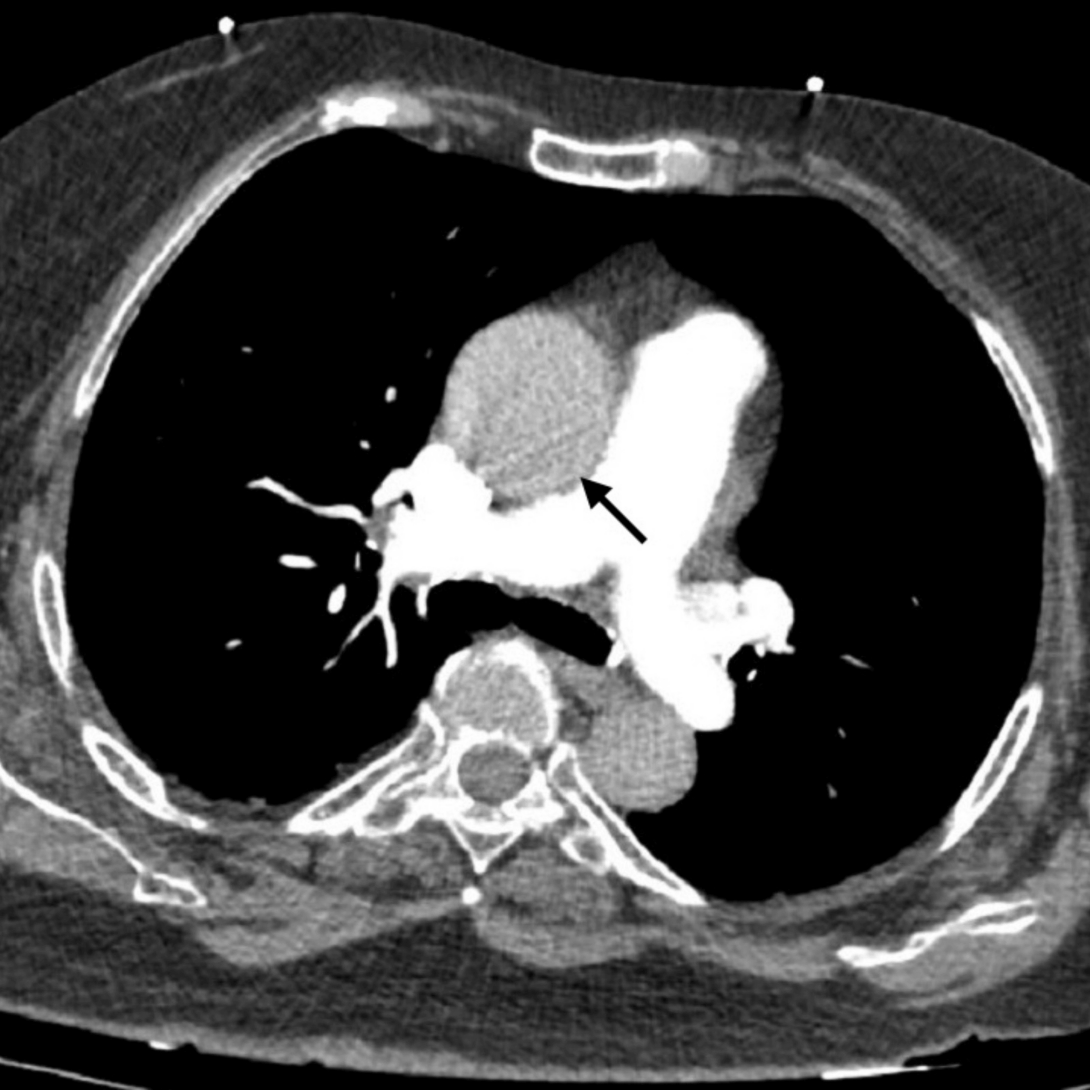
CT angiography of the chest showing mild dilatation of the ascending thoracic aorta (43 mm), as well as no filling defect in the major branches of pulmonary trunk. CT: computed tomography.
